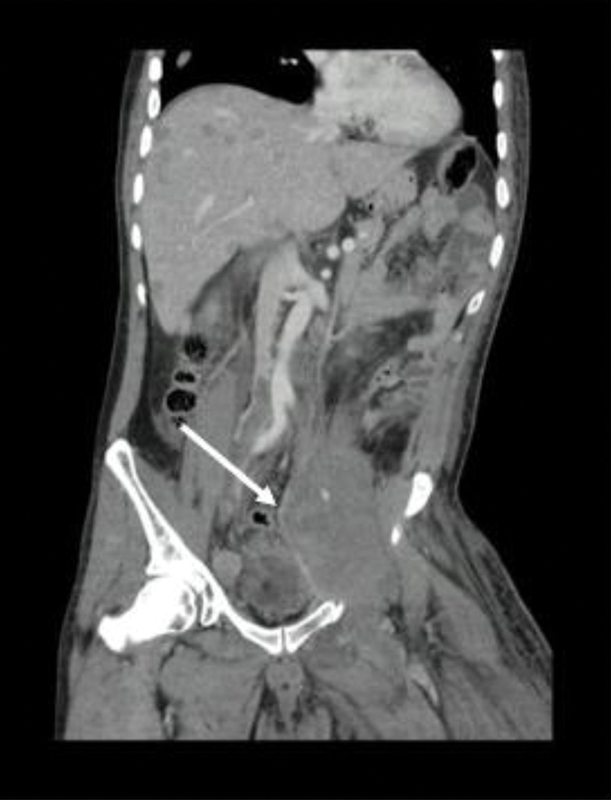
Thoracoabdominal CT in a coronal view, with a soft tissue mass infiltrating the left psoas muscle extending to the inguinal region (arrow).
